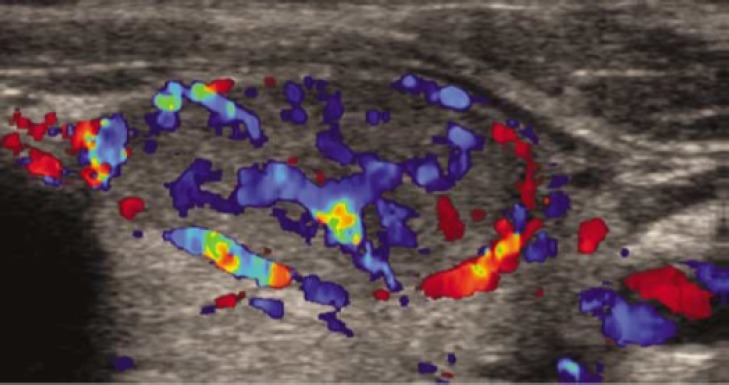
Preference central hypervascularity on color Doppler sonography as the most common finding in malignant nodules. There is marked intranodular blood flow and less significant perinodular blood flow
